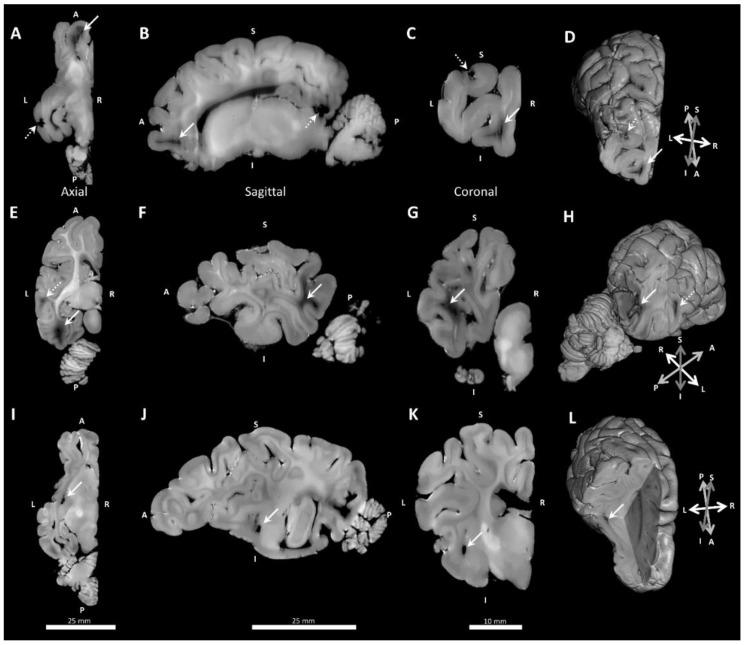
Example lesions in T1-weighted MRI images shown in orthogonal planes and with 3D rendering. (A–D) Frontal cortex white matter lesions (solid white arrows) and examples of susceptibility artefacts due to undissolved gas (stippled arrows). (E–H) Temporal lobe cortex lesion (stippled arrow) and occipital cortex white matter lesion (solid white arrow). (I–L) Deep cystic lesions (solid white arrows). Abbreviations: L, Left; R, Right; I, Inferior; S, Superior; A, Anterior; P, Posterior.
